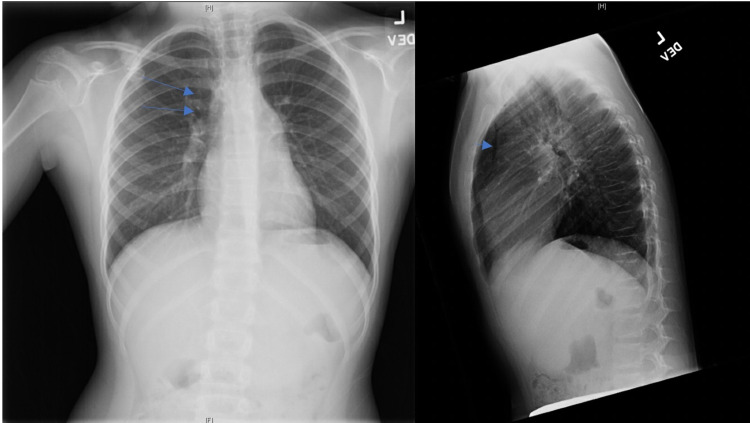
Follow-Up Anterior/Posterior and Lateral Chest X-ray There is a mild decrease in the size of the SPM noted in the anterior/posterior (left) and lateral (right) chest X-rays in comparison to that seen in Figure 1.
